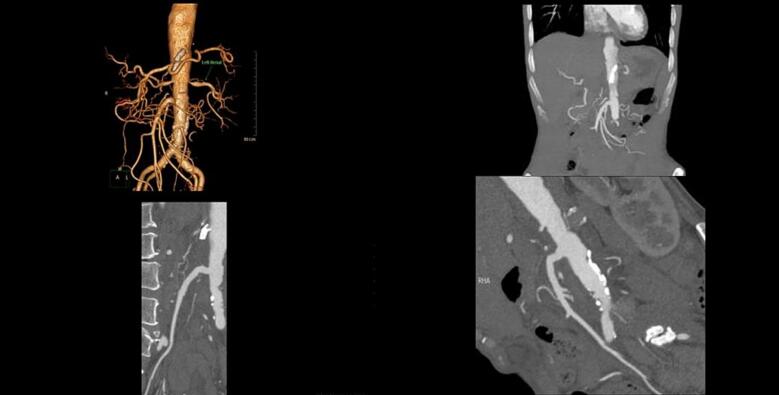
Abdominal CT angiography of patient one month after the surgery showing normal blood flow of the end-to-side anastomosis.
